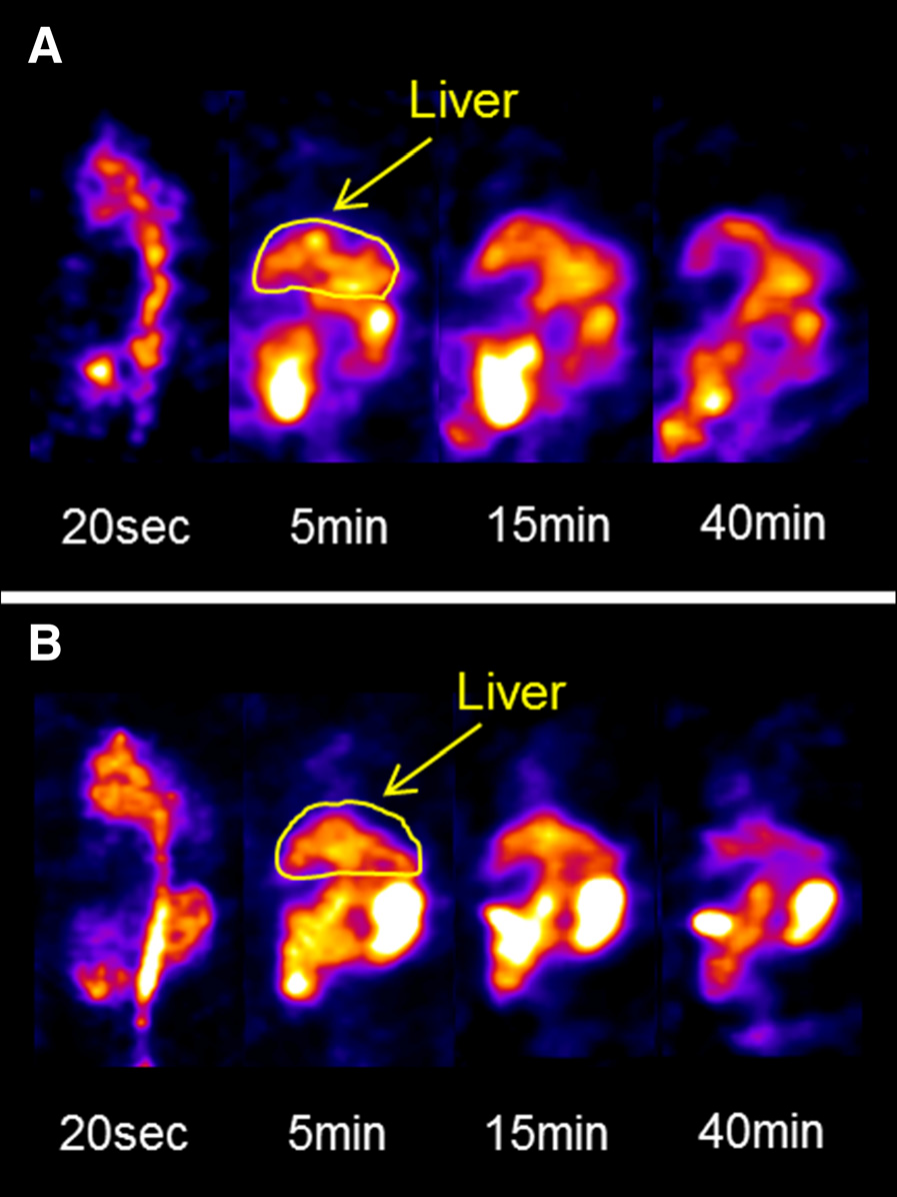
Dynamic SPECT images after
